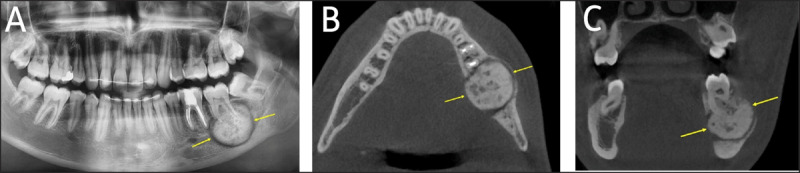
(A) Cementoblastoma. Panoramic radiograph of a periapical, sclerotic, sharply marginated lesion with a low-attenuation halo (arrows). The lesion is fused to the root of the tooth. (B, C) Cementoblastoma. Axial and coronal CT images showing a periapical, sclerotic, sharply marginated lesion with a low-attenuation halo. The lesion is fused to the root of the tooth and causes root resorption, loss of the root outline, cortical erosion, and obliteration of the periodontal ligament space (arrows).
